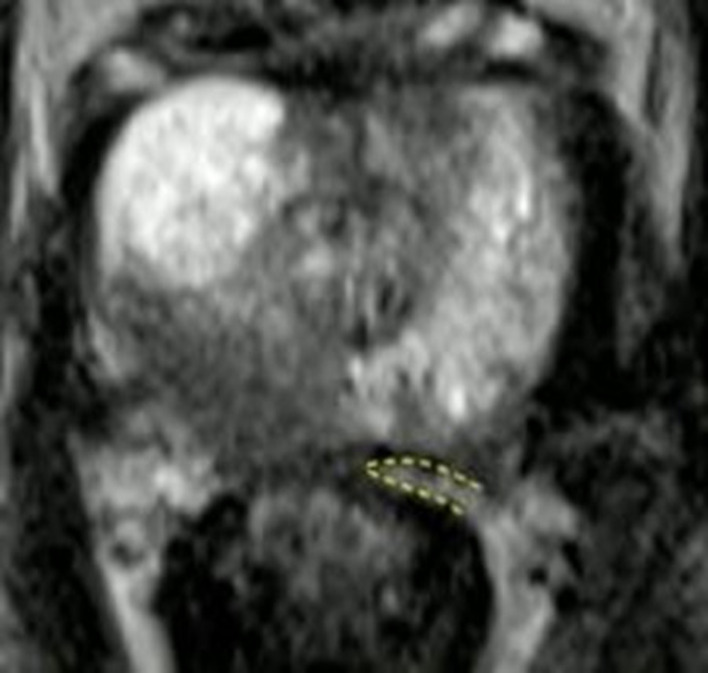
Recto-prostatic angle obliteration is seen in the axial sequences T2WI magnetic resonance imaging. The disappearance of the adipose angle depends on the tumor burden. DWI, diffusion-weighted imaging.
